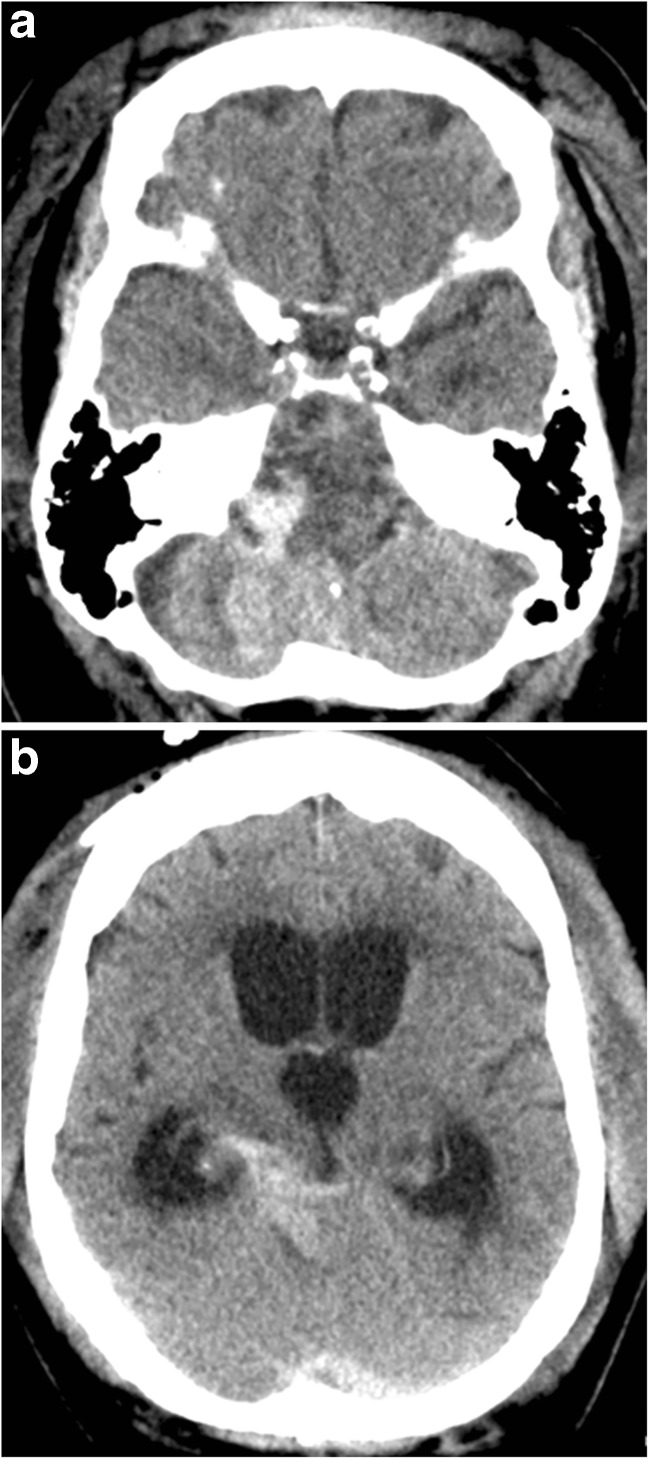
a and b Axial CT images of the brain demonstrate an extra-axial hemorrhage around the brainstem and in the posterior fossa, causing an acute obstructive hydrocephalus
